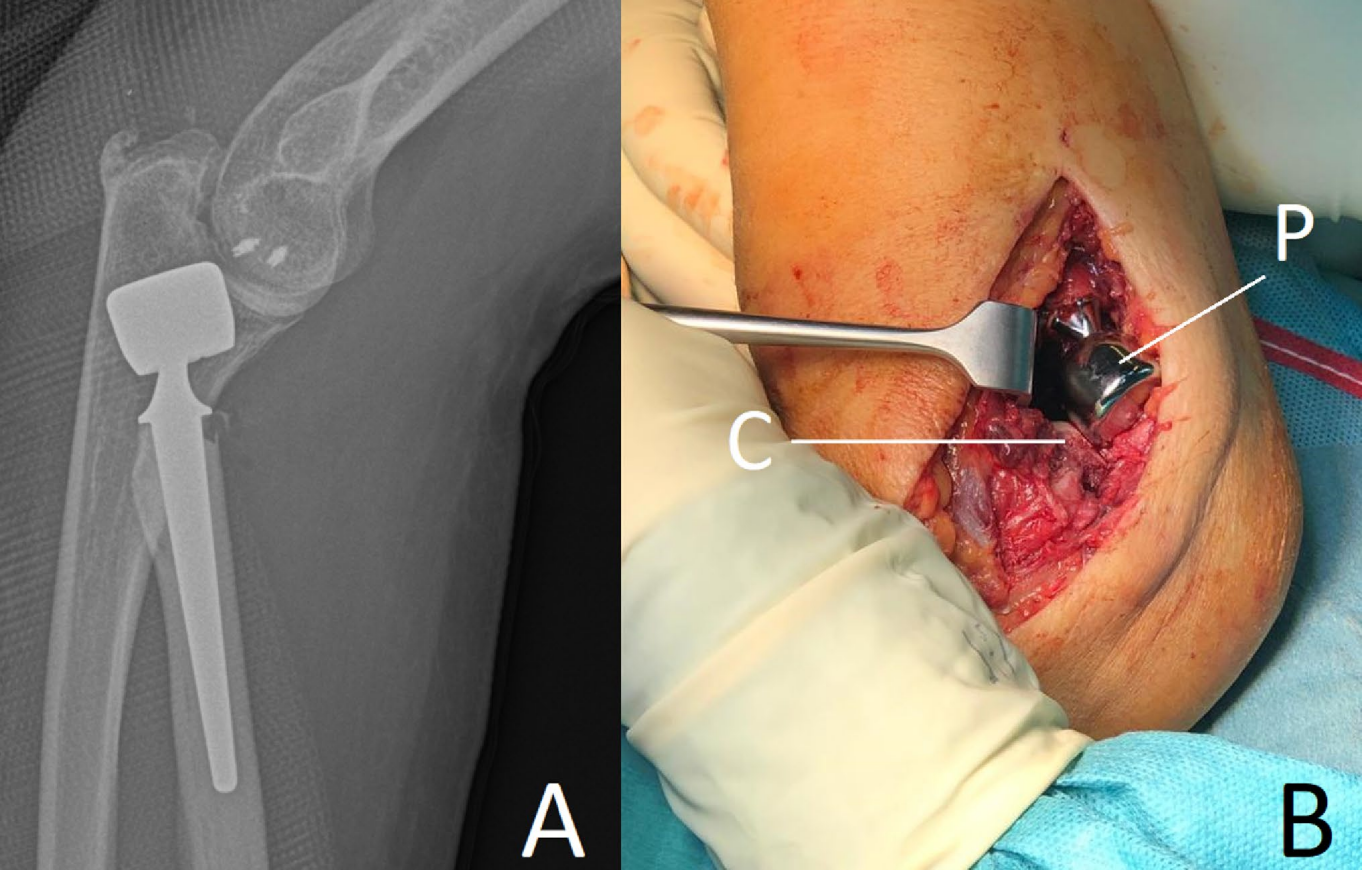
Example of high-grade overlengthening, where the immense pressure between the prosthesis and the capitulum leads to the implant being pushed out dorsally. a Lateral X-ray. b Intraoperative findings. *C* capitulum, *P* prosthetic implant
